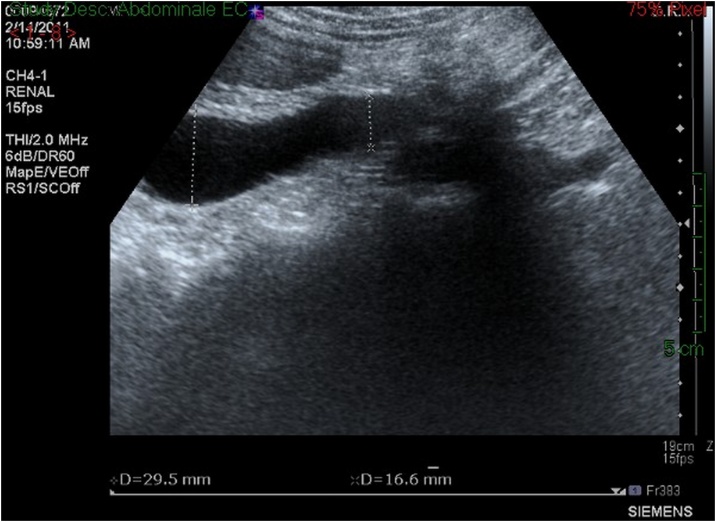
Renal ultrasound of patient 2 showing severe right-sided hydronephrosis.
